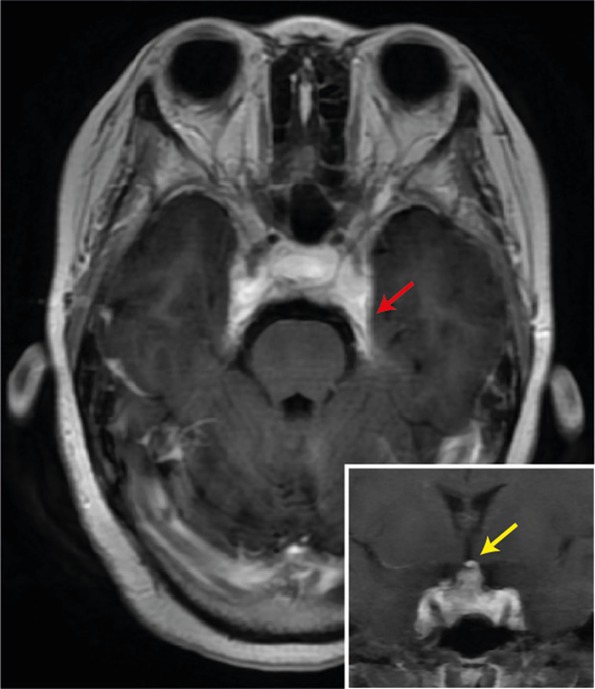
Imaging feature. T1‐weighted contrast‐enhanced MRI showed a heterogeneous enhancing sellar mass. This lesion invaded the bilateral cavernous sinuses (red arrow, axial view) and protruded into the suprasellar cistern (yellow arrow, coronal view, inset).
